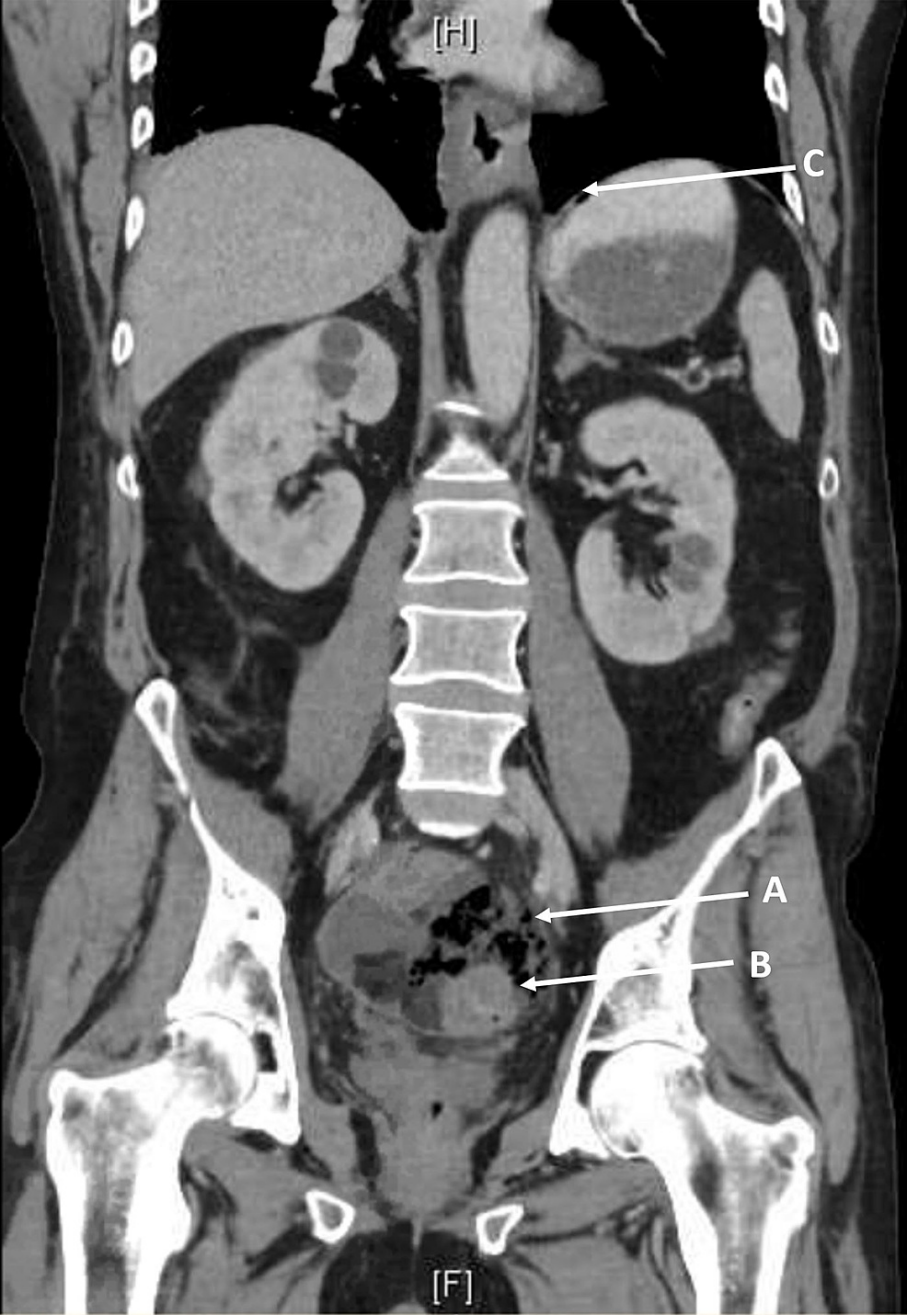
Coronal CT Abdomen and Pelvis Initial CT abdomen and pelvis on presentation to Emergency Department with A = extraluminal gas showing perforation, B = thickened rectosigmoid junction, and C = gas under diaphragm
